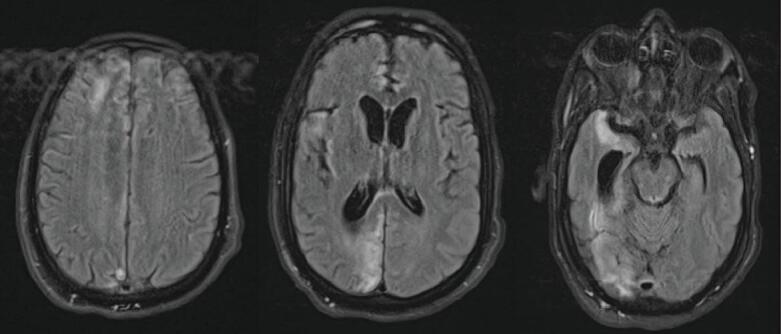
MRI Head two months after discharge T2-weighted MRI Head showing evolving multifocal encephalomalacia with right hemisphere volume loss and enlargement of right lateral ventricle.
